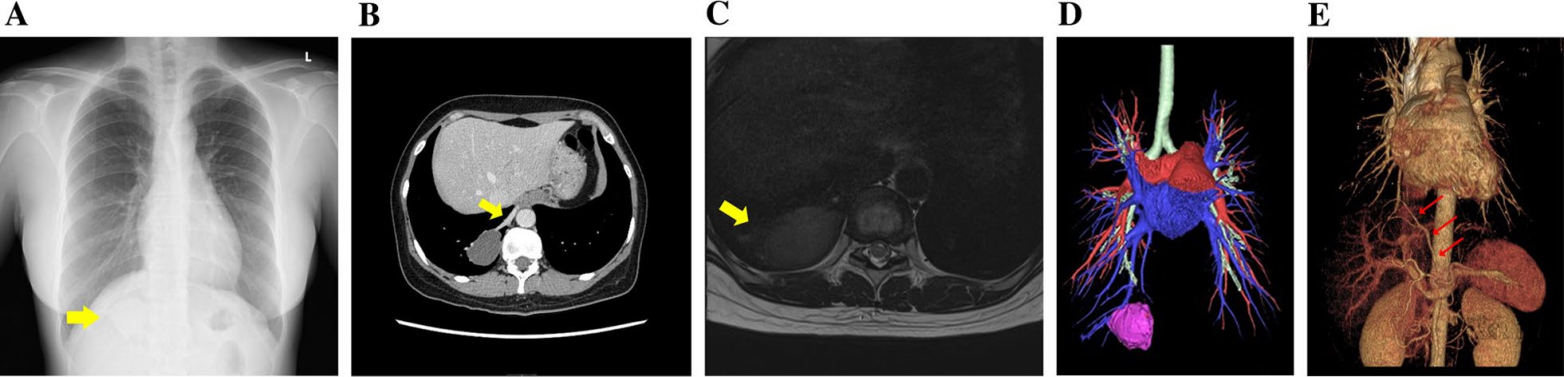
A Chest X-ray displayed a solid nodule (yellow arrow) in the right lower lung field. B Enhanced chest computed tomography displayed a solid mass of 4.5 × 3.1 cm in the right S10 area, accompanied by an aberrant artery (yellow arrow) supplying blood to the mass. C Chest magnetic resonance imaging displayed low intensity on T2-weighed image in the mass area (yellow arrow). D Three-dimensional reconstruction image shows the involvement of pulmonary veins (blue) in the mass (pink), while neither the pulmonary artery (red) nor the bronchus (light green) was involved. E Three-dimensional reconstruction image shows that the aberrant vessel (red arrows) inflowing into the mass is found to branch off from the celiac artery
